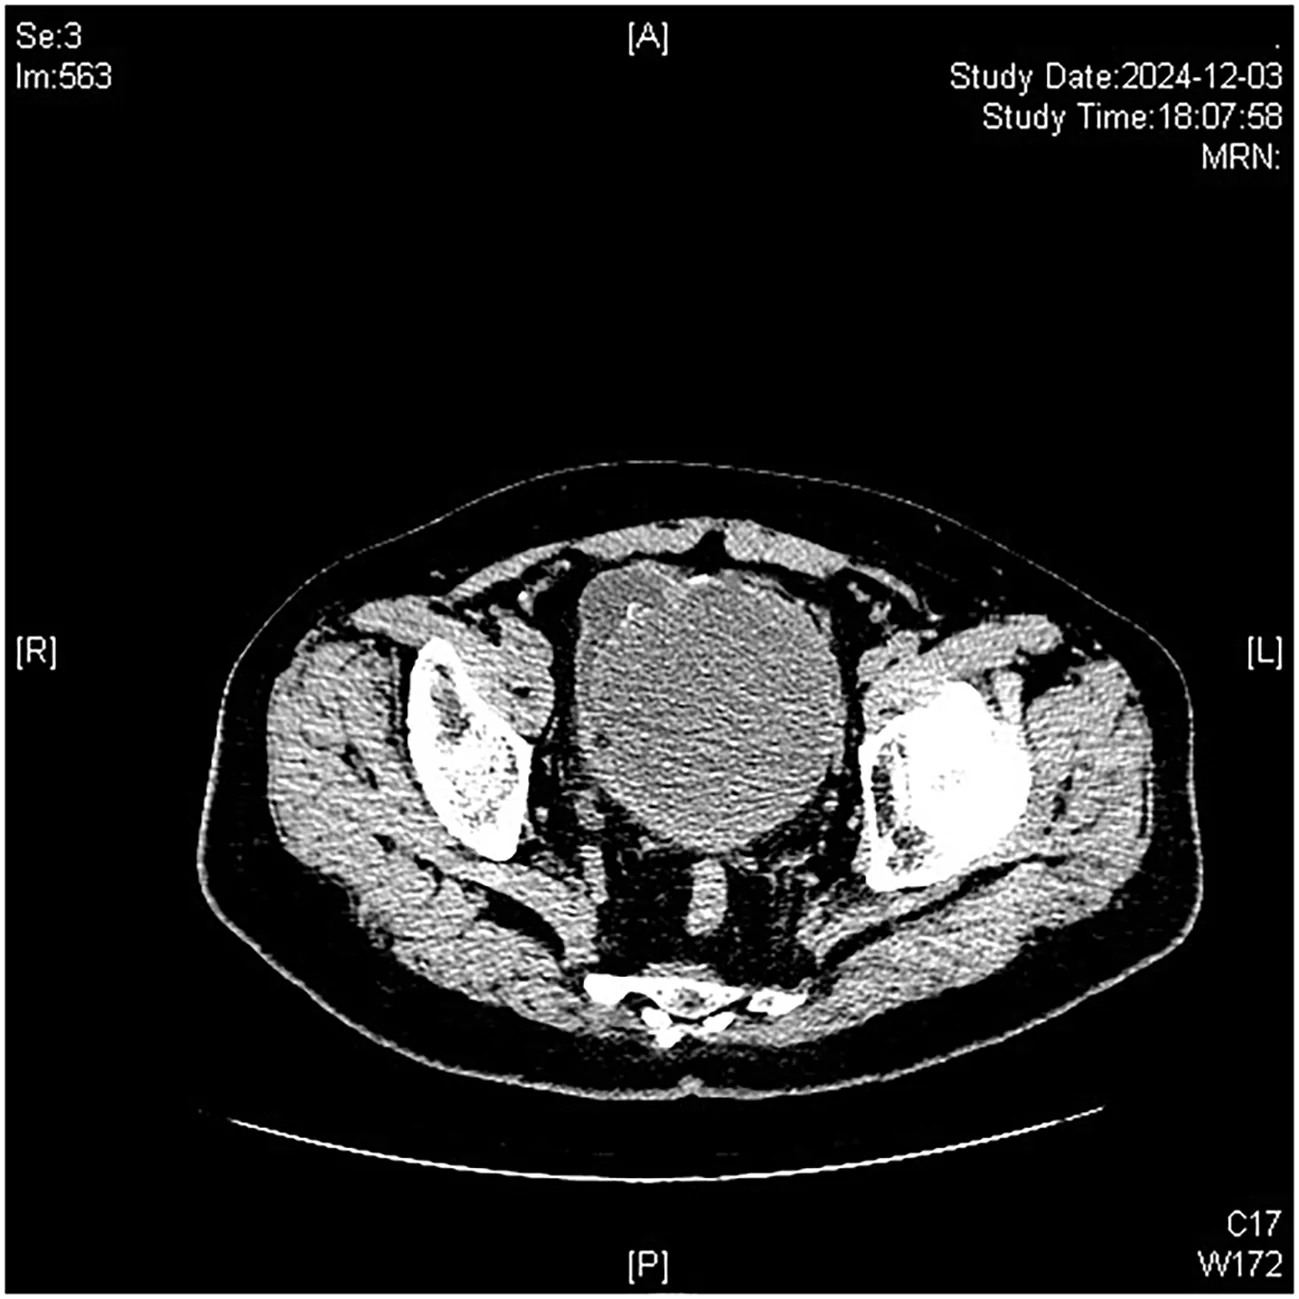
Postoperative abdominal CT scan showing a bladder diverticulum with calcification (arrow) measuring 5.2 × 2.4 cm.
